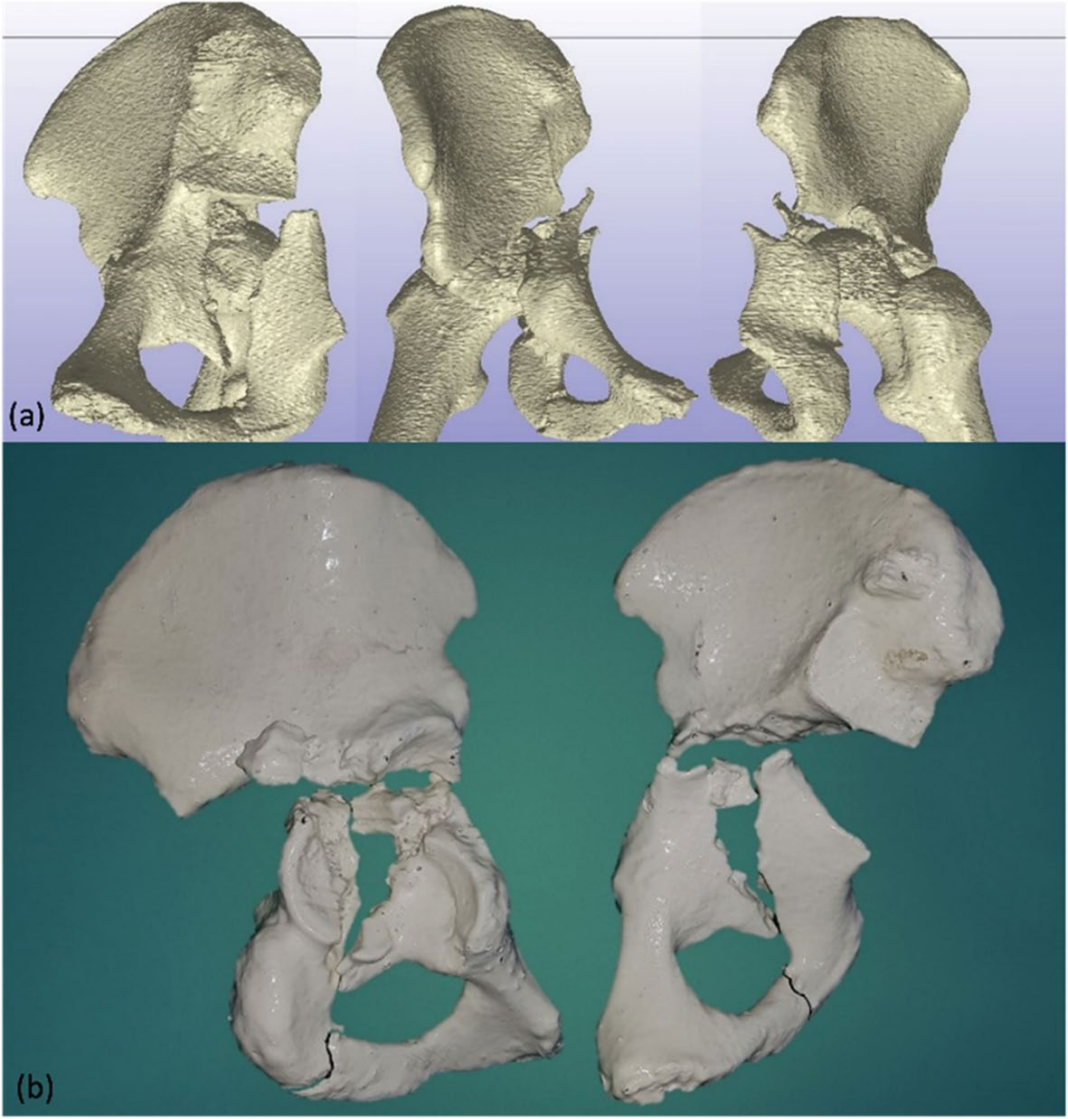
a Reconstruction of images from the CT scan of case of ‘T-shaped’ acetabular fracture and (b) 3D printed models of the same demonstrating the orientation of the fracture
